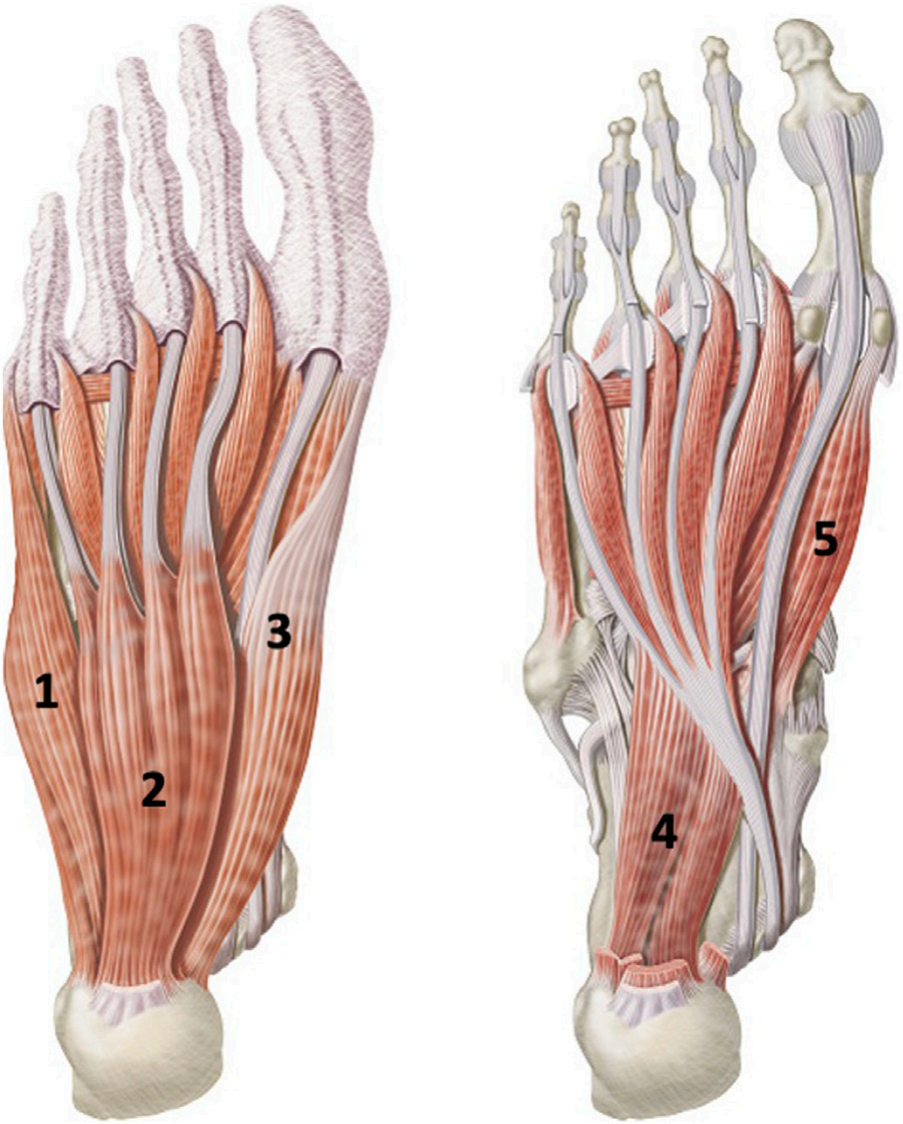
Five plantar intrinsic foot muscles measured by ultrasound imaging. 1. abductor digiti minimi, 2. Flexor digitorum brevis, 3. Abductor hallucis, 4. Quadratus plantae, and 5. Flexor hallucis brevis. Images purchased via iStock by Getty Images, Calgary, Alberta Canada.
